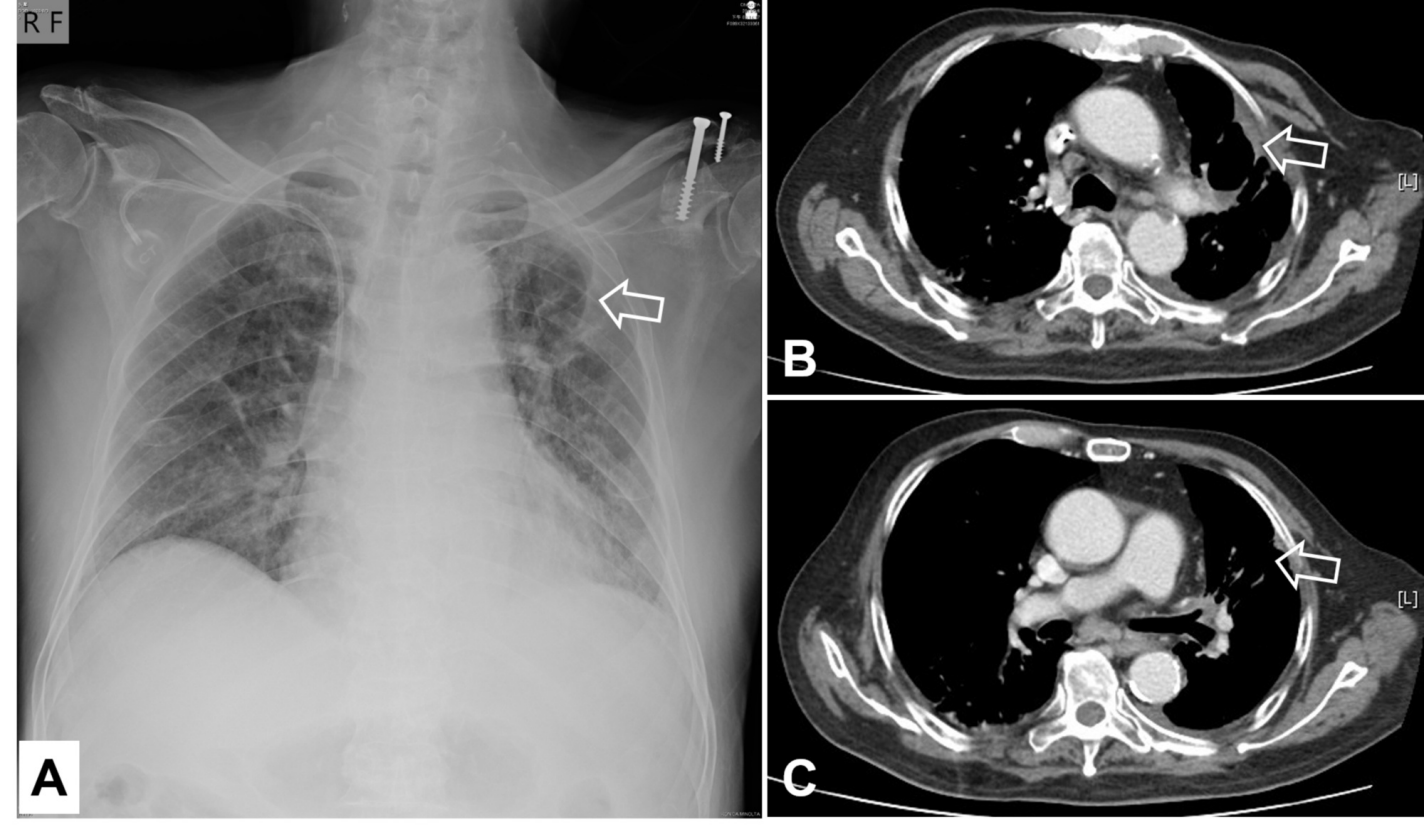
Radiological findings of nearly complete remission of the original malignant lesion, as compared with Figure 1: A. Chest X-ray film, September 6, 2019; B and C. CT scan, September 9, 2019 Arrows: Targets of interest
